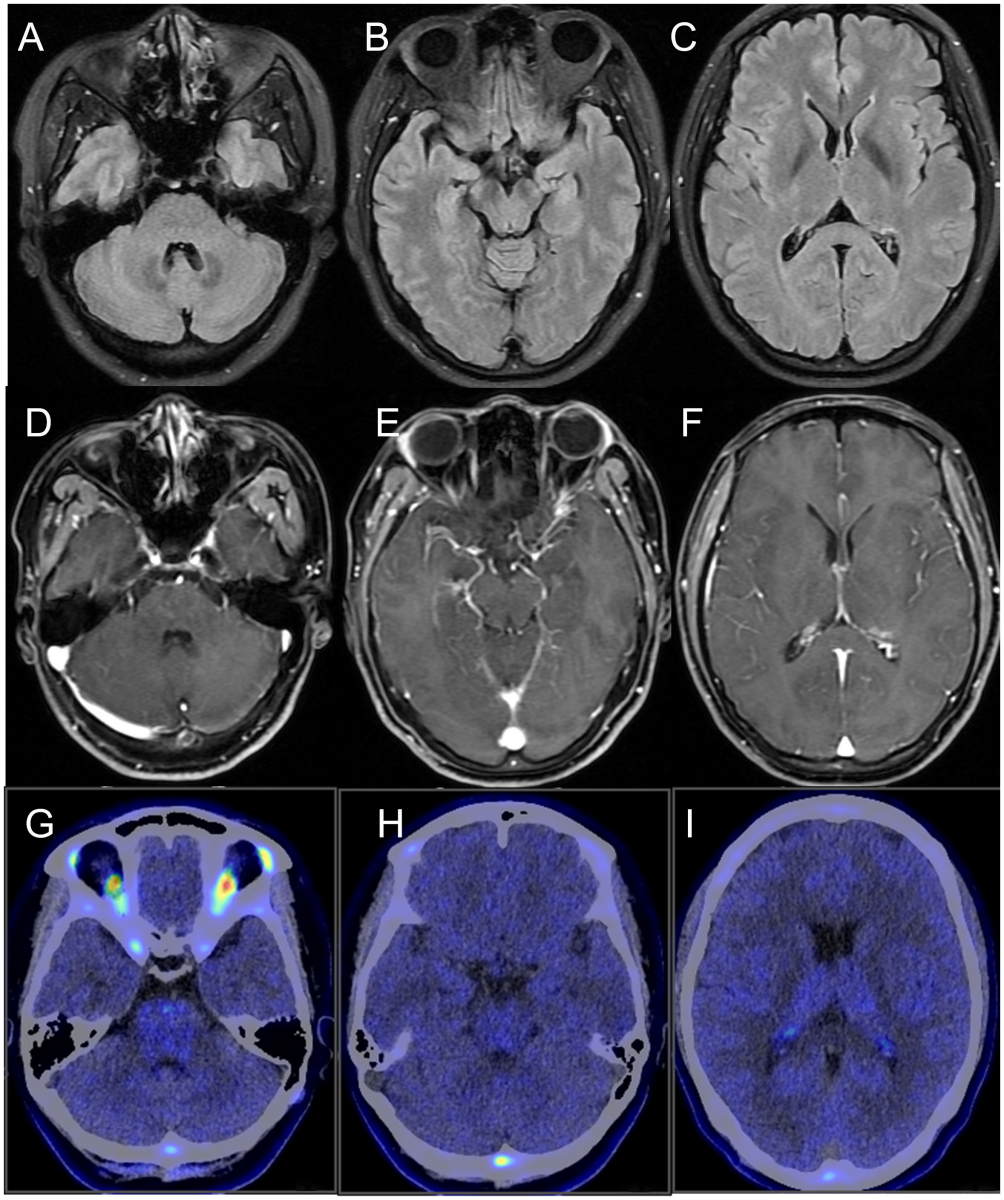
Neuroimaging of the patient. (A-C) brain MRI fluid-attenuated inversion recovery sequence; (D-F) brain MRI T1-weighted contrast-enhanced sequence; (G-I) brain 18F-DPA714 PET/CT. No abnormalities were detected.
